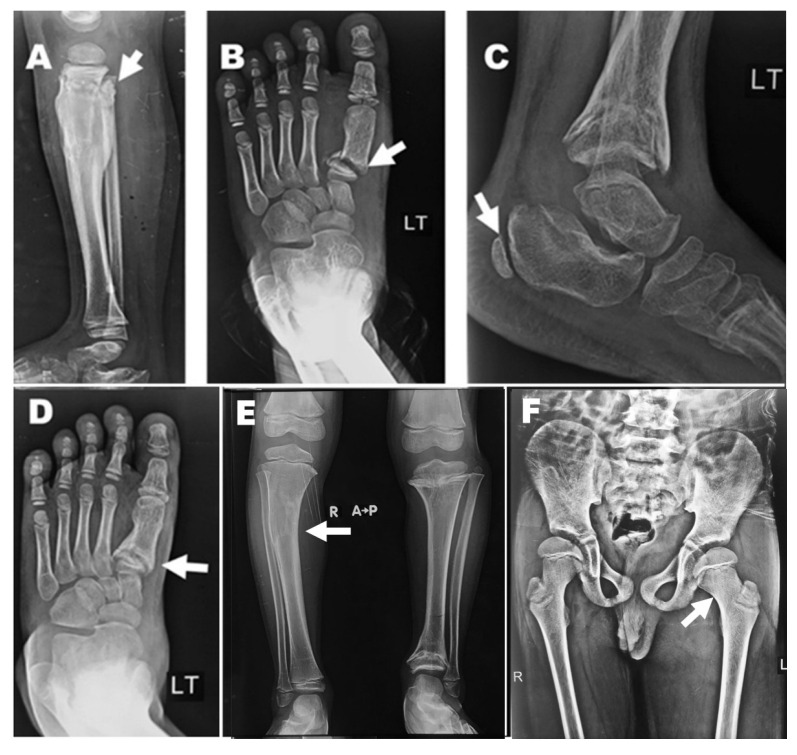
Orthopedic radiographs, the arrows show hypertrophic bone callus formation (A) left 1st metatarsal fracture (B), left calcaneal fracture (C), imperfect bone consolidation of 1st metatarsal fracture (D), right bowed legs with shorter left leg (E), deformity of the left hip where there is an increased angle between the femoral neck and femoral shaft (F).
